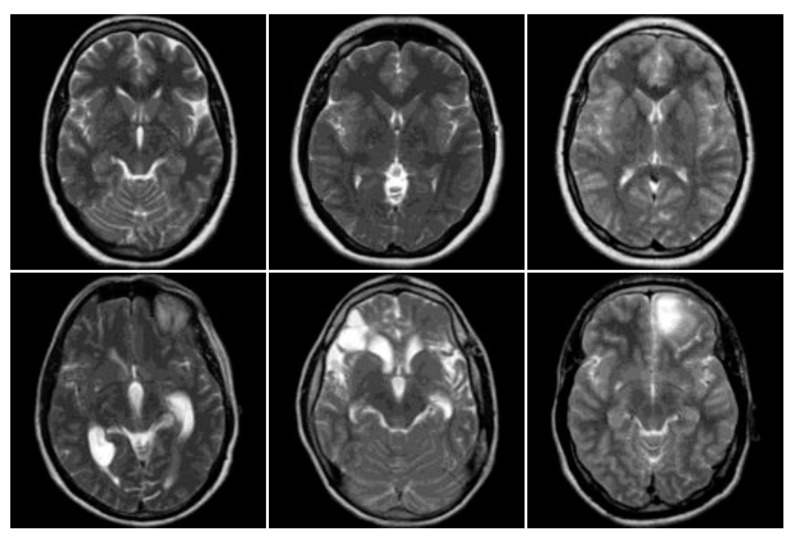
MRI brain images from the collected dataset.
